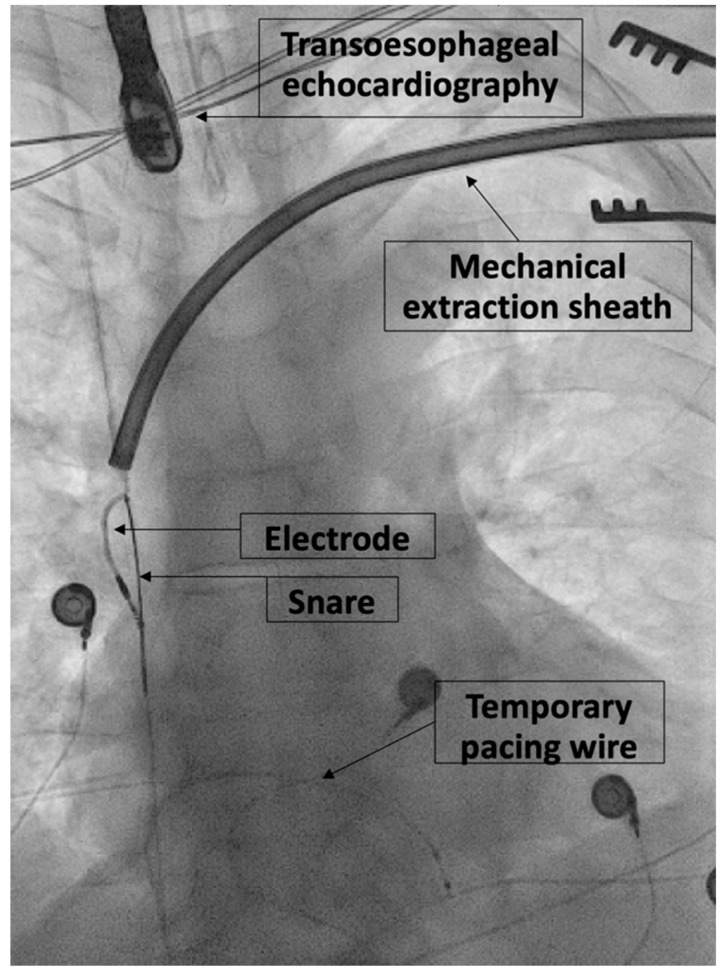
X-ray during lead extraction with combined superior and femoral approach.
